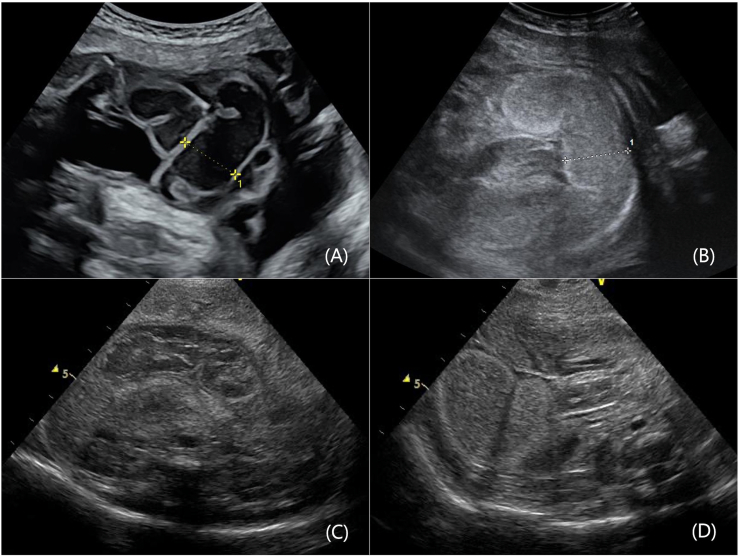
Prenatal ultrasound examination of the fetal abdomen shows intestinal dilatation of 24 mm and thickening of the intestinal wall during a prenatal checkup at 34 weeks of pregnancy (A), and intestinal dilation worsening of the diameter to 26 mm and the high intestinal brightness at 36 weeks and 5 days of pregnancy (B). Postnatal abdominal ultrasound examination shows dilated bowel loops and thickening of the intestinal wall (C, D), whereas meconium is detected from the sigmoid colon to the rectum without ascites (D).
